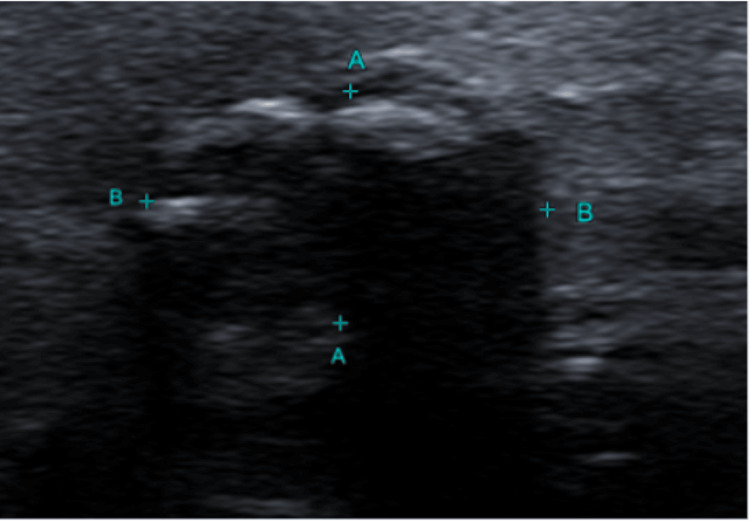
Case 1: thyroid ultrasound showing left thyroid lobe nodule (TIRADS 4) (distance A: 7.1 mm; distance B: 12.2 mm) TIRADS 4: Thyroid Imaging Reporting and Data System Category 4
